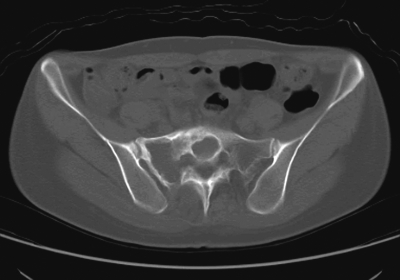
A 32-year-old female with fibrous dysplasia in the sacrum. More than half of the sacral body was occupied by the tumor, which invaded the sacral canal to a slight degree. Computed tomography showed good bone formation of the curetted wall 1 year after the operation.
